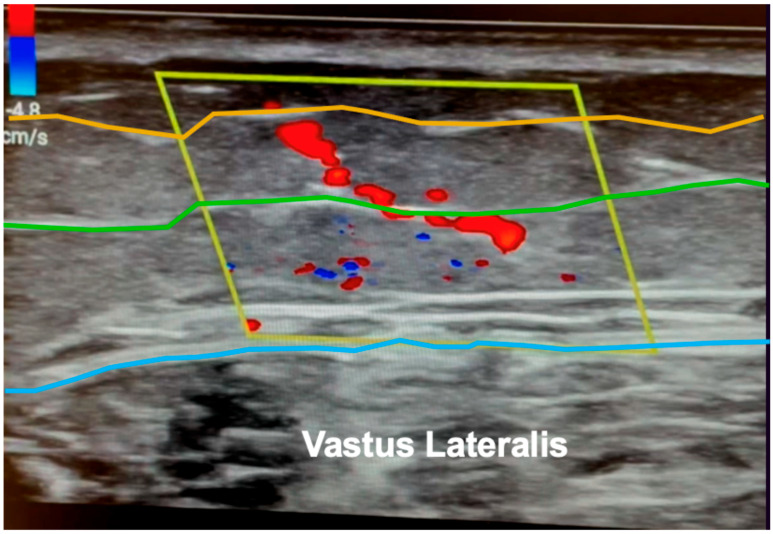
Color duplex ultrasonography (CDU) of an anterolateral thigh perforator. The deep fascia (*blue line*) corresponding to the traditional plane of dissection, superficial fascia (*green line*) corresponding to the thin plane of dissection, and subcutaneous plane (*orange line*) corresponding to the superthin plane of dissection are highlighted. The course of a perforator traversing the subcutaneous tissue and superficial fascial planes is easily visualized in *red*. Imaging performed by senior author A.A.S.
